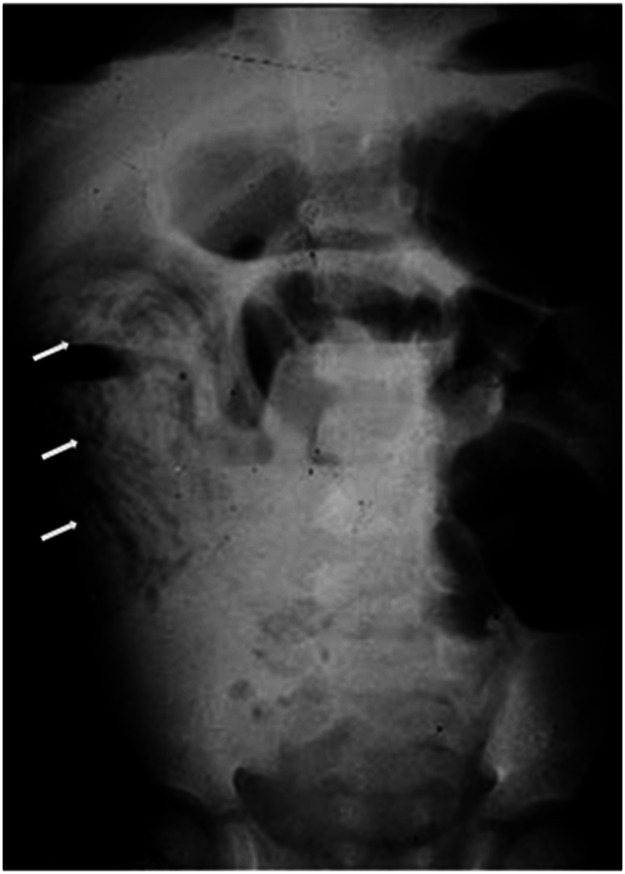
Abdominal plain abdominal X-ray showing intestinal obstruction by Ascaris (arrows).
